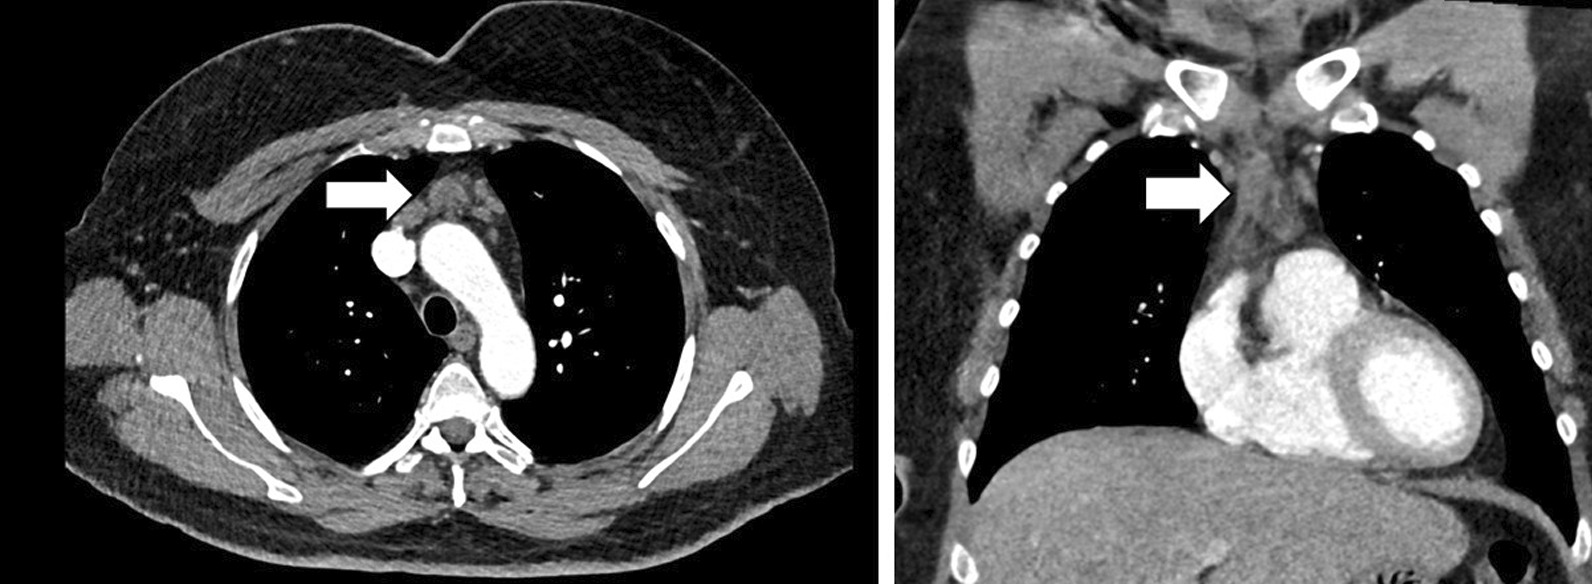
Contrast enhanced CT of the chest was performed using thin section reconstructions. The patient was given intravenous contrast with iopamidol. A 1.8 cm prevascular lymph node was seen on axial and sagittal views
